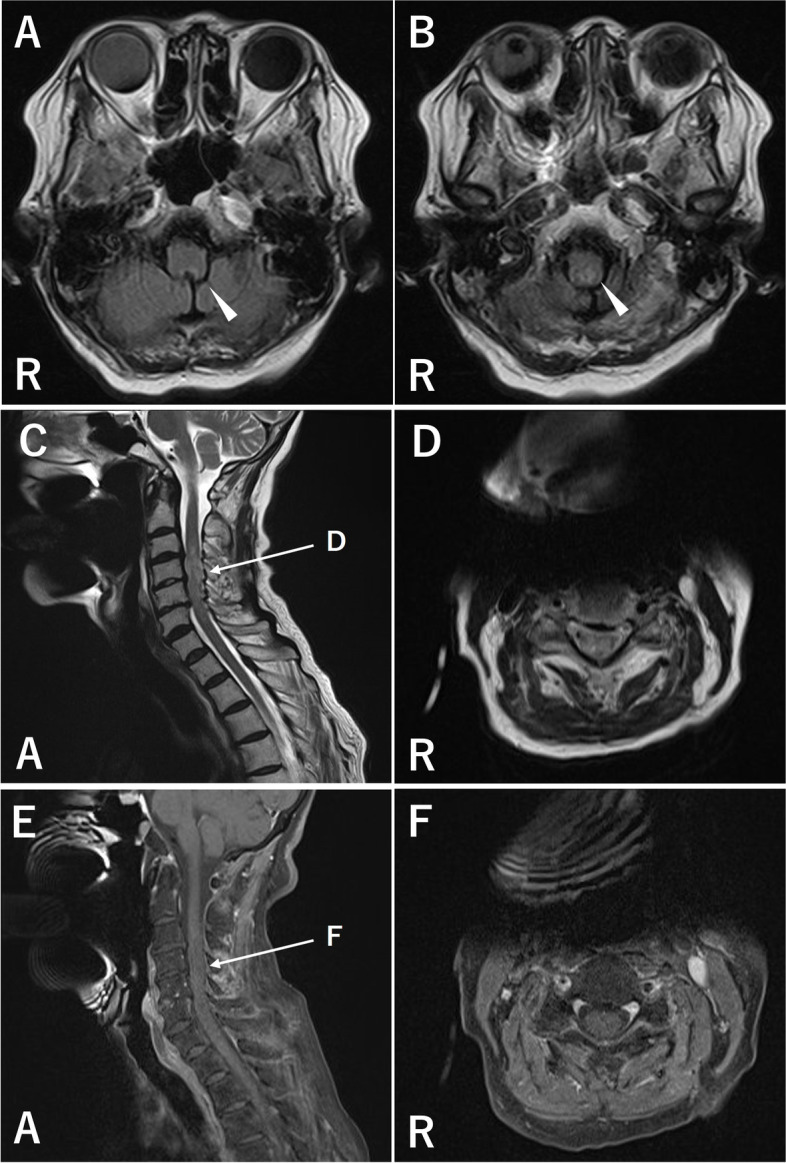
MR imaging of the brain and cervical cord. Axial FLAIR brain MRI revealed a high-intensity lesion in the dorsal part of the medulla oblongata (A, B; arrowheads). On T2-weighted cervical MRI, a high-intensity lesion existed in the C3–6 cervical cord (C, D), while slight enhancement was observed in those corresponding areas on gadolinium-enhanced T1-weighted images (E, F)
